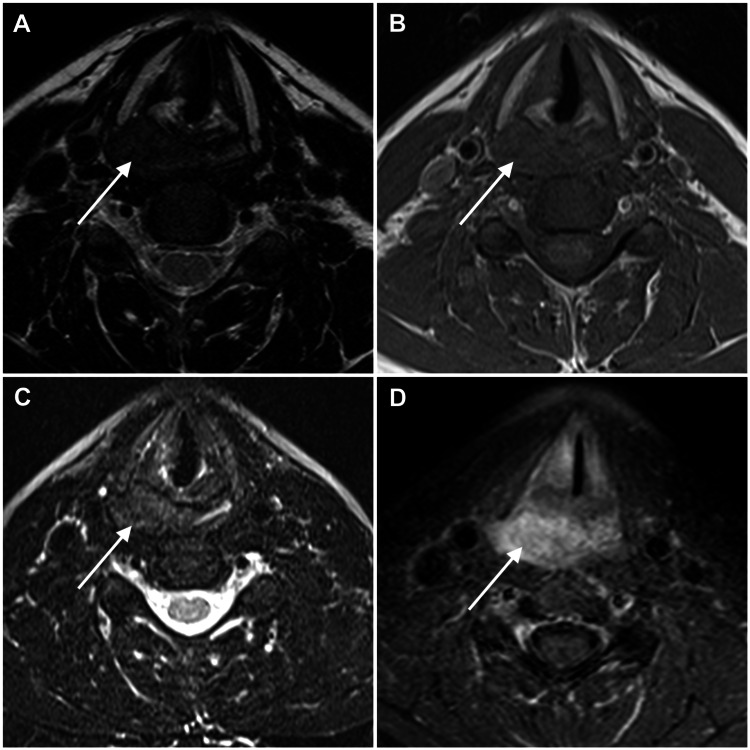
Magnetic resonance imaging of the lesion (white arrow). Both axial T2-weighted image (T2WI) (A) and fat-suppressed T2WI (C) showed a slightly hyperintense lesion to the muscle. On axial T1-weighted image (T1WI), the lesion (B) showed homogeneous isointense signal intensity compared to muscle. On axial T1 post-contrast image, the lesion had homogeneous enhancement.
